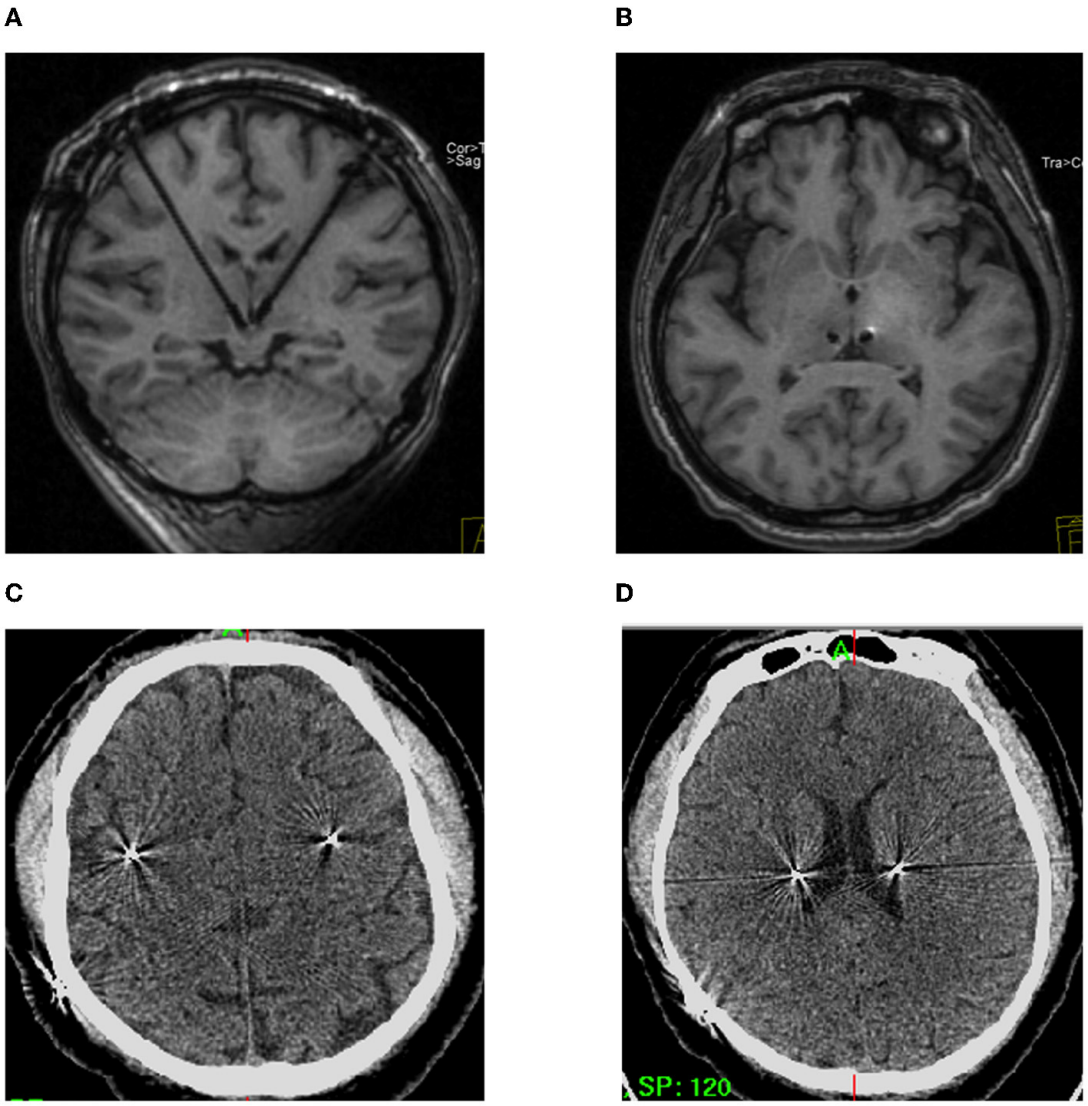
Safety of the frontal trajectory of the surgical path to the LHb. (A, B) No hematoma or ischemia as confirmed by intraoperative MRI. (C, D) Post-operative CT within 72 h on different planes.
